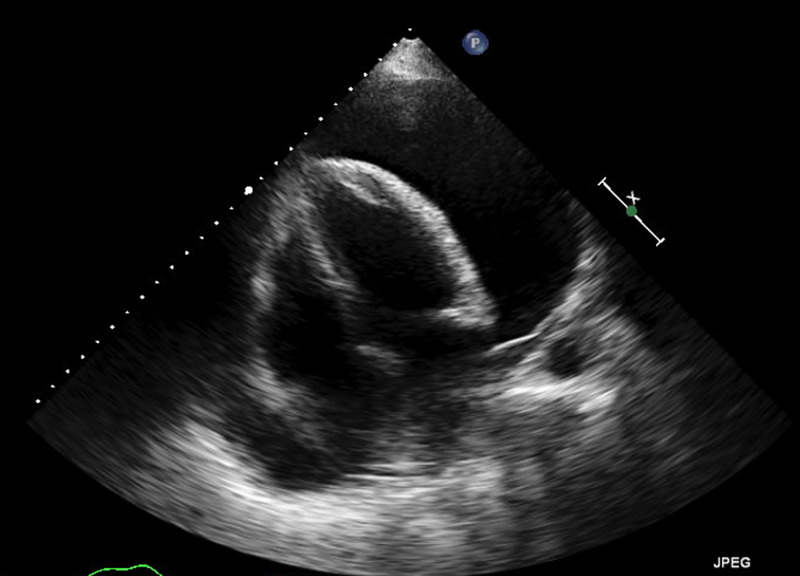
Transthoracic echocardiogram shows large pericardial effusion.
